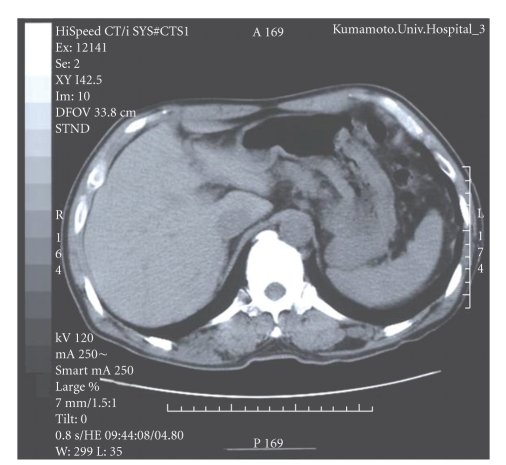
Normalization of CT density of the liver after 6-month discontinuation of amiodarone (63 Hounsfield units).
